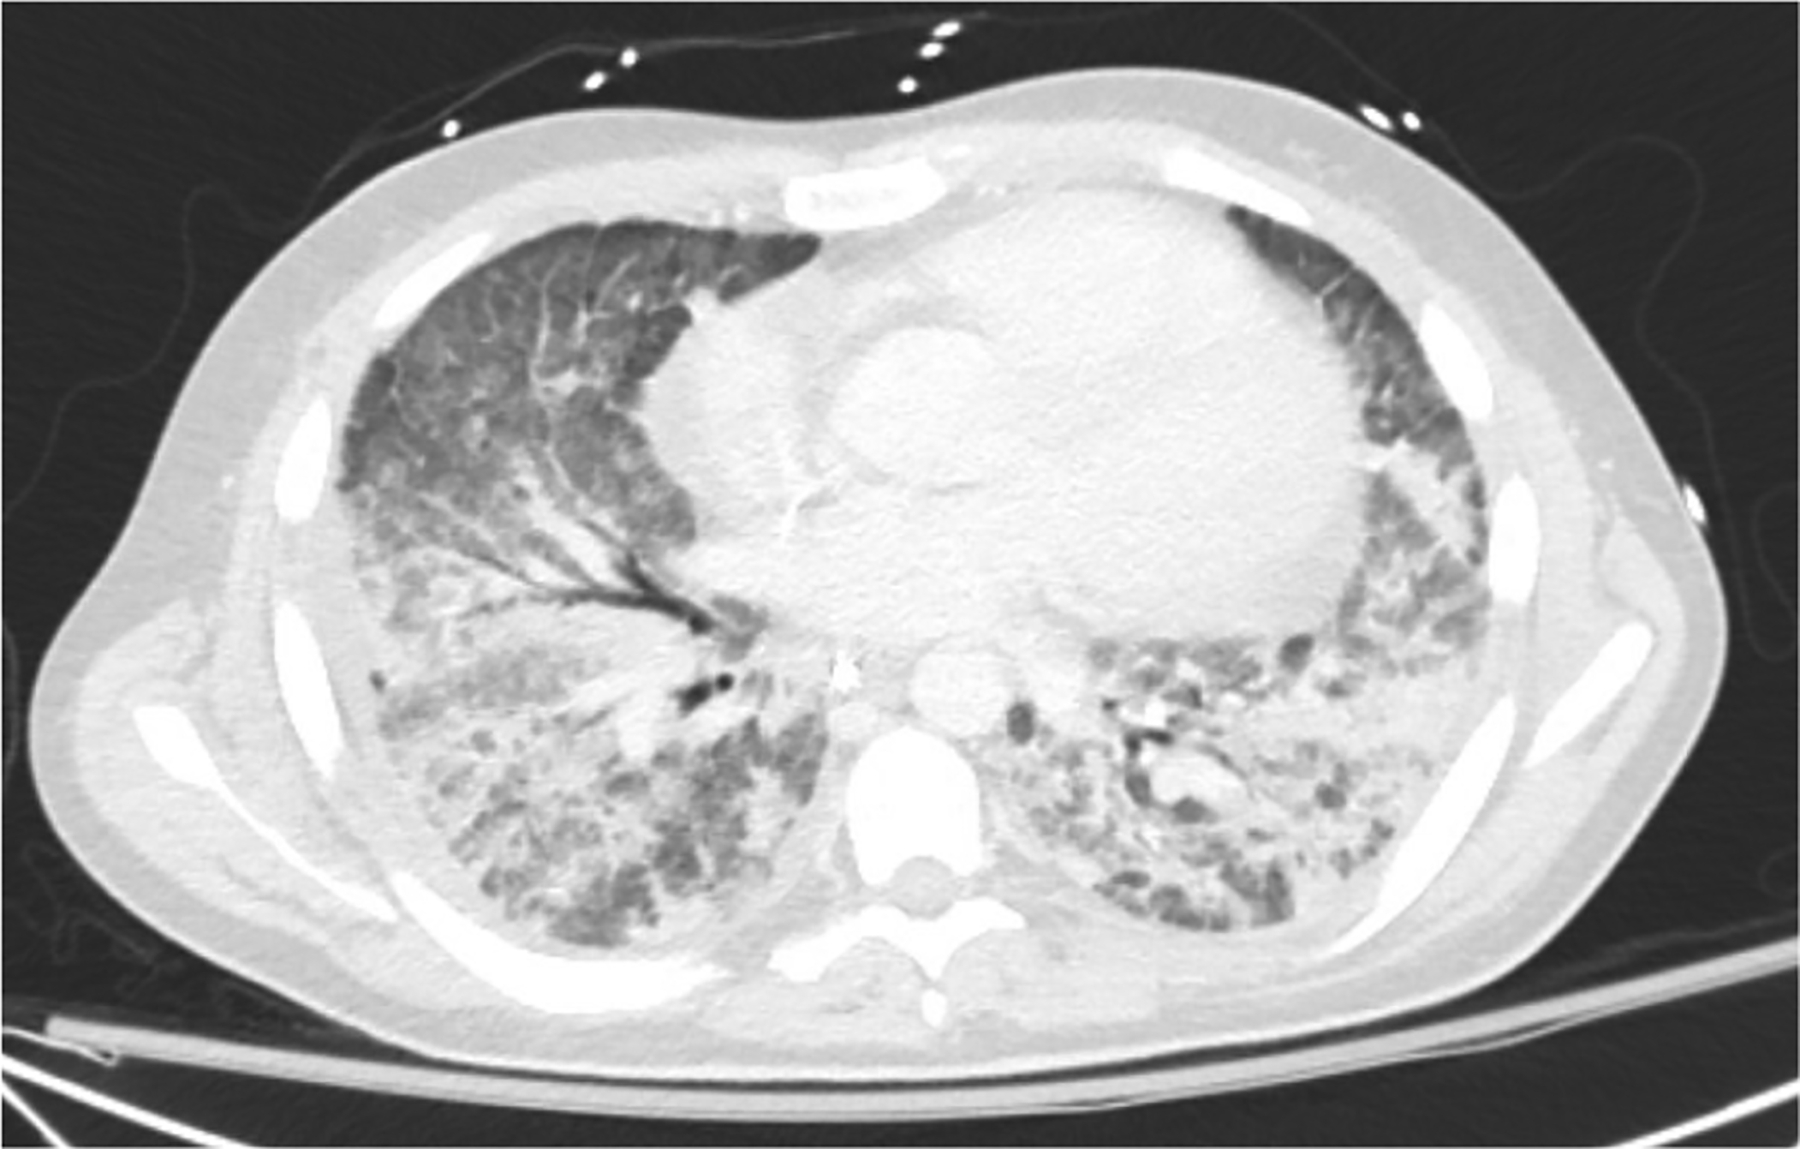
Axial computed tomography (CT) image, corresponding with hospital day 35 in Figure 1, demonstrating extensive bilateral airspace consolidation and areas of ground glass attenuation.
